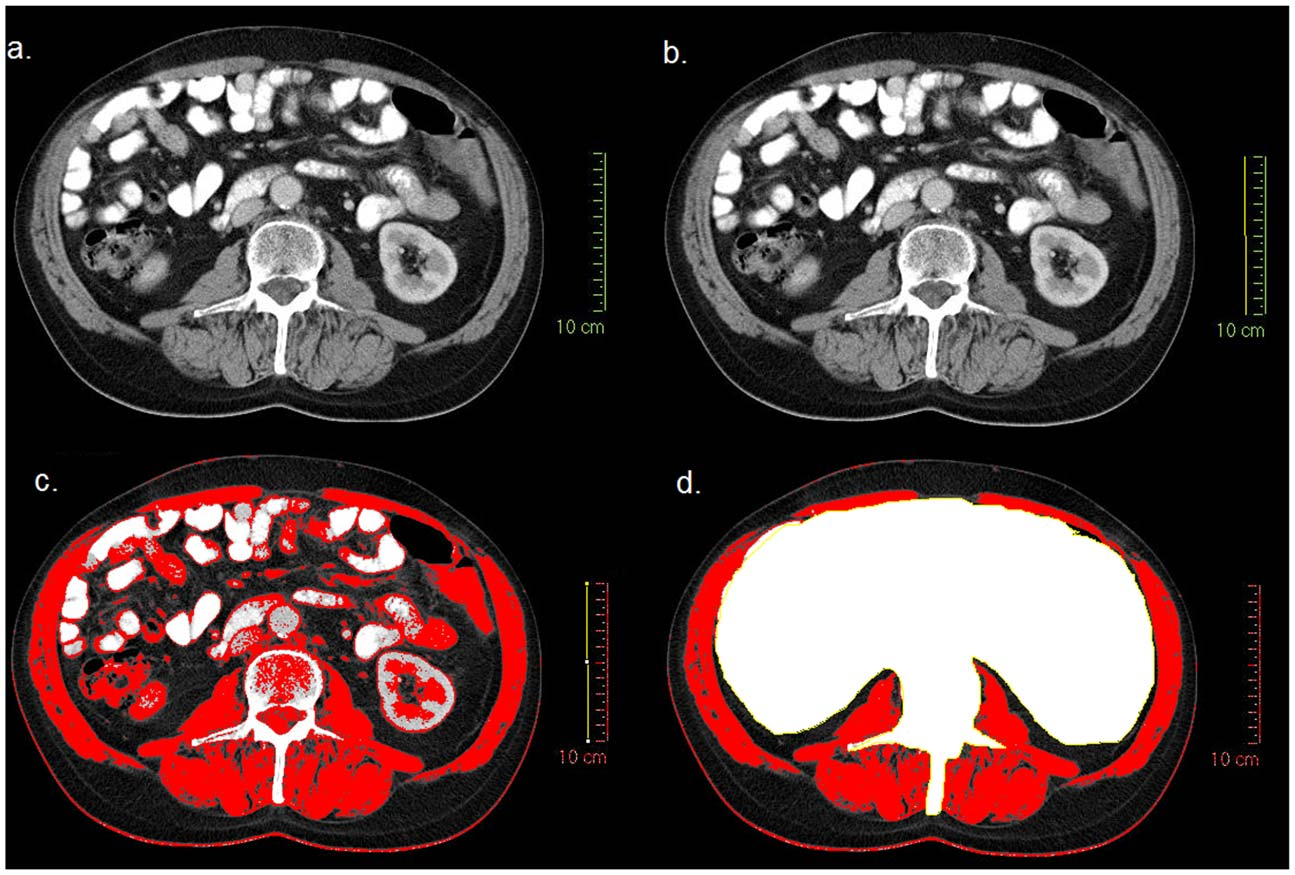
An example of CT image analysis using NIH ImageJ software. (a) the original CT image in JPEG format, (b) the scale is set using a known distance (10 cm) from the original CT image, (c) skeletal muscle thresholds (−29 to+150 HU) are applied, (d) the abdominal contents and L3 vertebrae are cropped and the skeletal muscle cross sectional area calculated in cm^2^.
